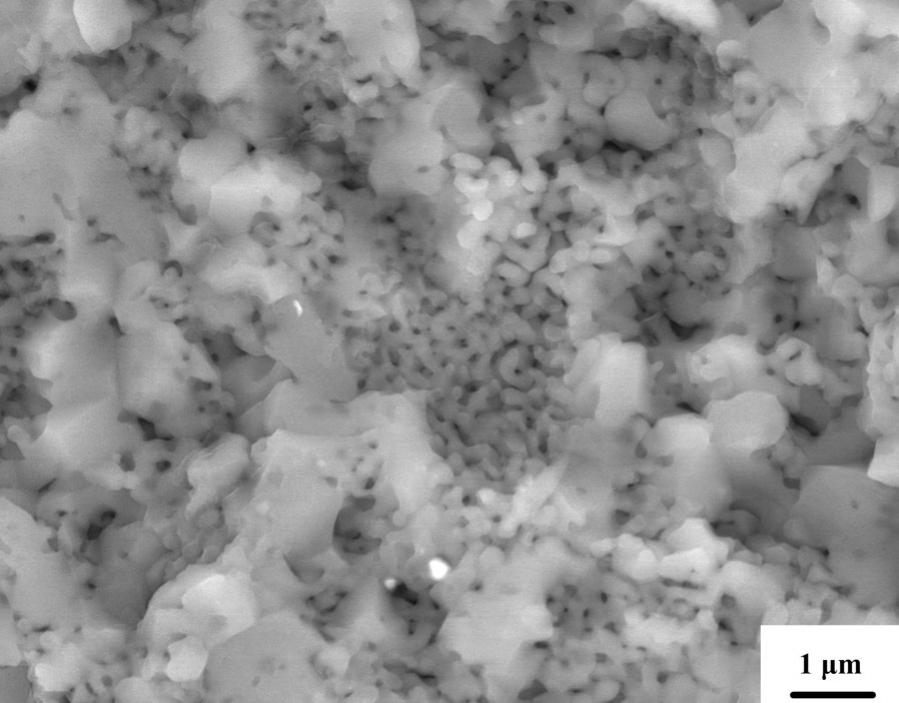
X-ray patterns of the alumina nanopowder sintered by SPS at 1400 °C
